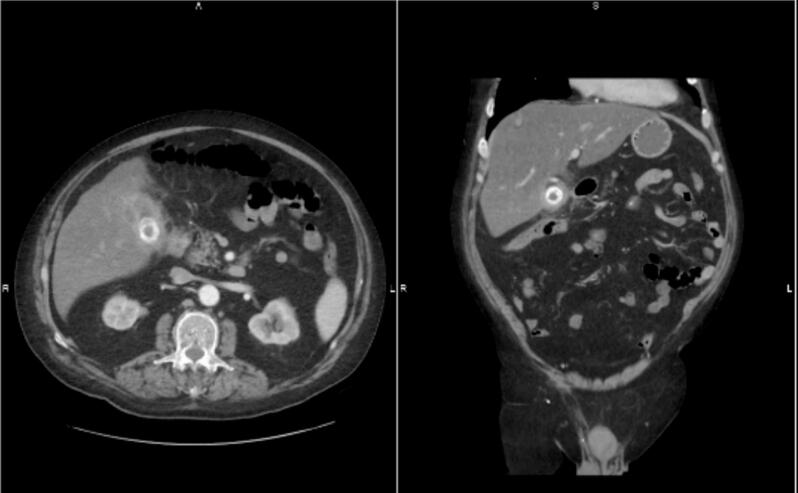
Patient 2's CT scan of the abdomen and pelvis showing a large gallstone within the gallbladder.
